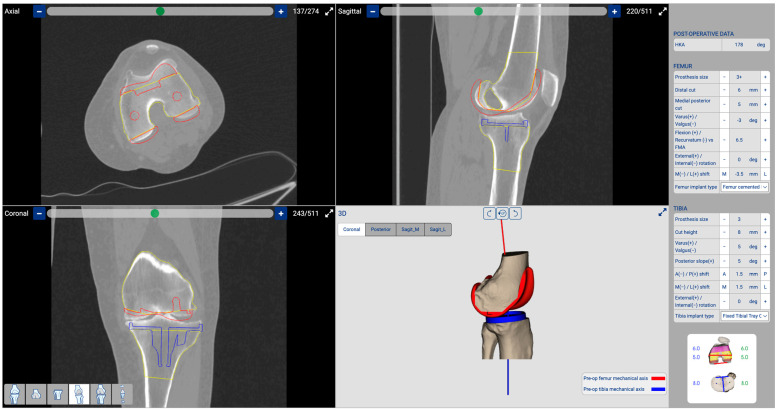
Three-dimensional planning: The software enables us to conduct three-dimensional planning based on a CT scan. To preserve the native LDFA, we planned a distal femoral cut at 2.5° valgus with a bone thickness of 6 mm. The posterior femoral cut was set at 0° of rotation with a bone thickness of 5 mm. The tibial cut was planned with a varus of 3.5° and a PTS of 8.5°. Additionally, we determined the optimal component sizes, selecting a femoral component size 3+ and a tibial component size 3. In red is represented the position of the planned femoral component, while in blue is the position of the tibial component.
